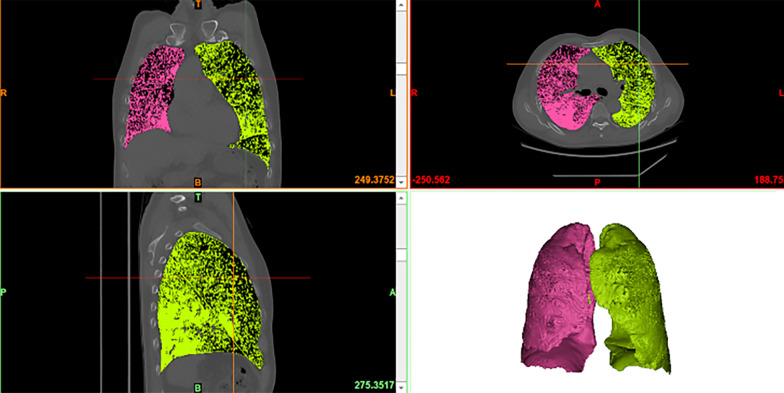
Coronal, axial and sagittal CT images and the FLV (CT threshold, − 910 HU to − 600 HU)
